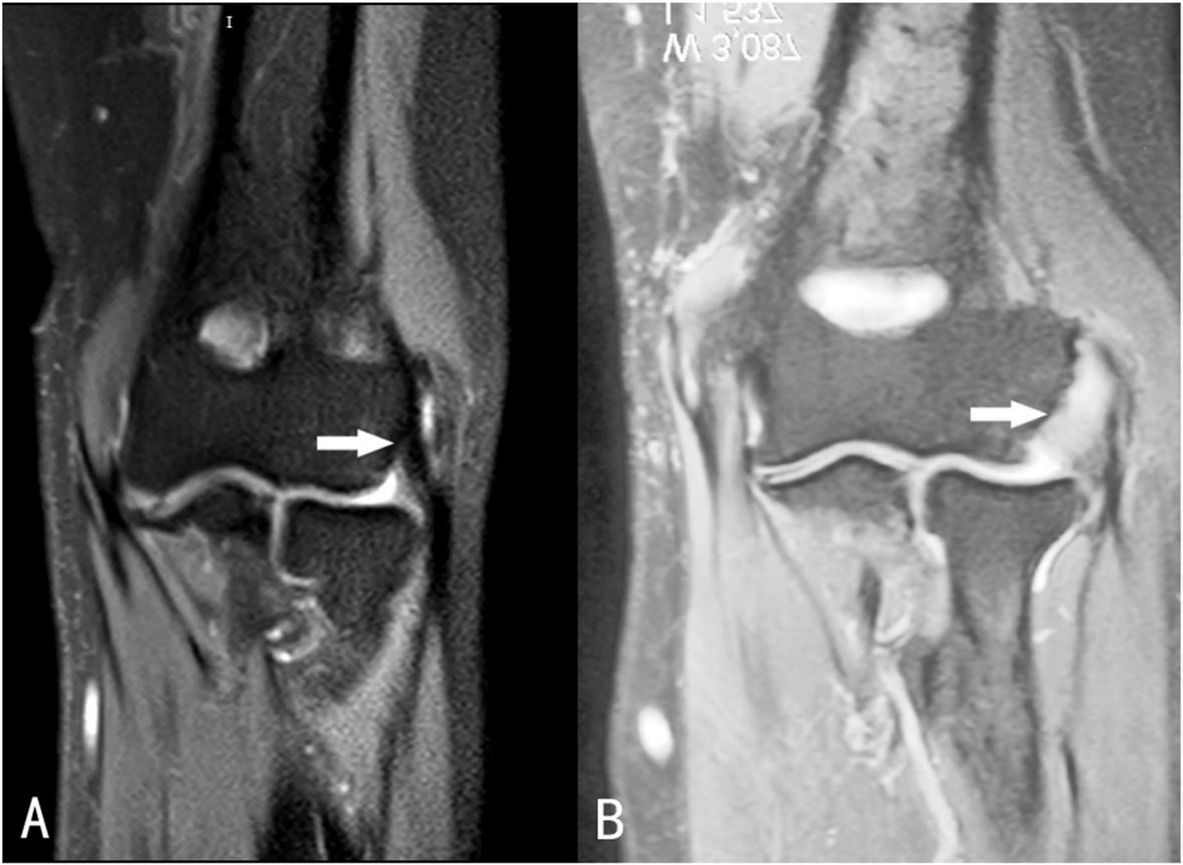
Examples of MRI findings on the LCL. A 53-year-old female, MRI showed normal low intensity signal of the LCL. B 49-year-old male, MRI detected abnormal higher intensity signal of the LCL (white arrow)
